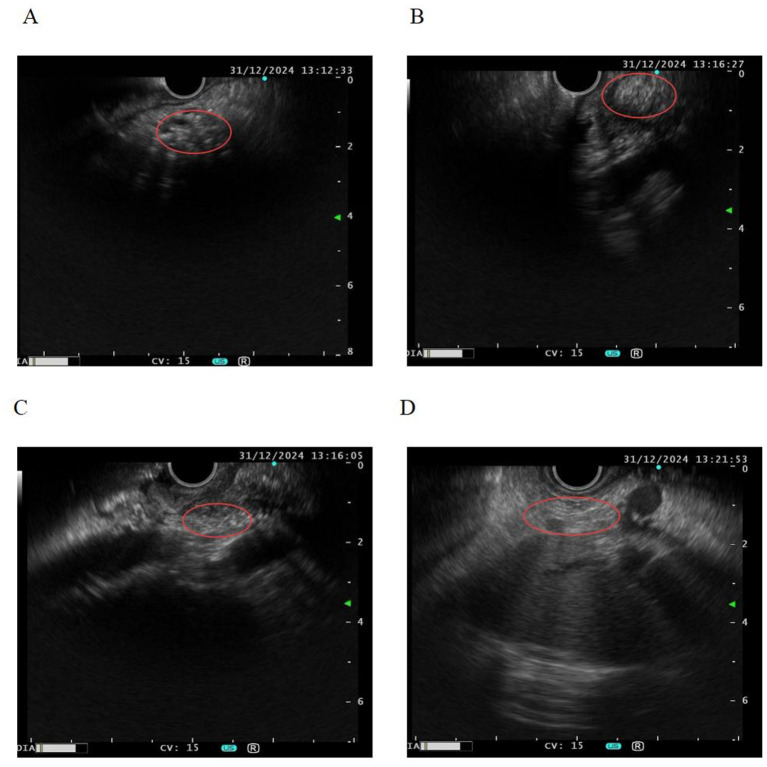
Endoscopic ultrasound. The volume of the pancreatic body and tail is significantly atrophic, with the parenchymal echo showing poor homogeneity. The head of the pancreas presents as short-stick, strand-like, and lobular hyper-echoic structures, with marked calcification and a slender pancreatic duct. (A) Head of the pancreas (atrophy and calcification in the pancreatic head region). (B, C) Calcification is present in the sulcal region of the pancreatic head. (D) Pancreatic body and tail (marked atrophy of the pancreatic body and tail).
